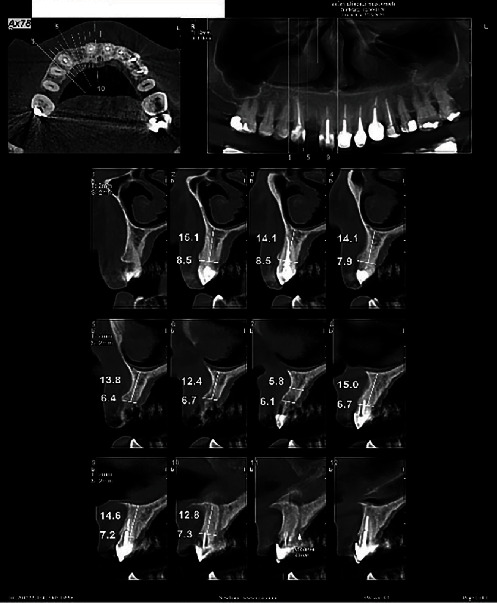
Postoperative CBCT scan of a patient. CBCT, cone-beam computed tomography.
